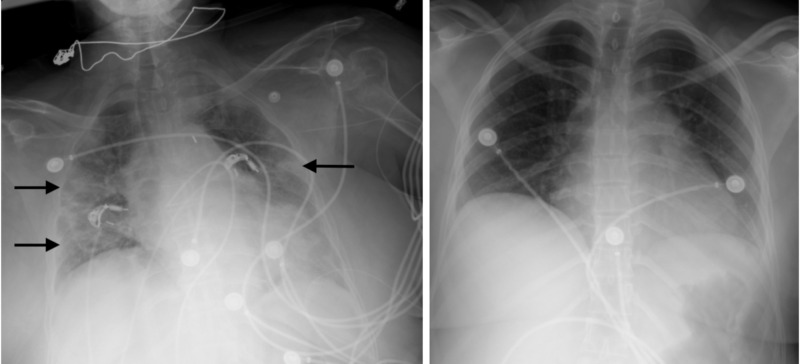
Chest x-ray on presentation for Ms. A (left) depicting diffuse bilateral infiltrate (arrows) vs. chest x-ray on presentation for Ms. B (right) without any significant findings
